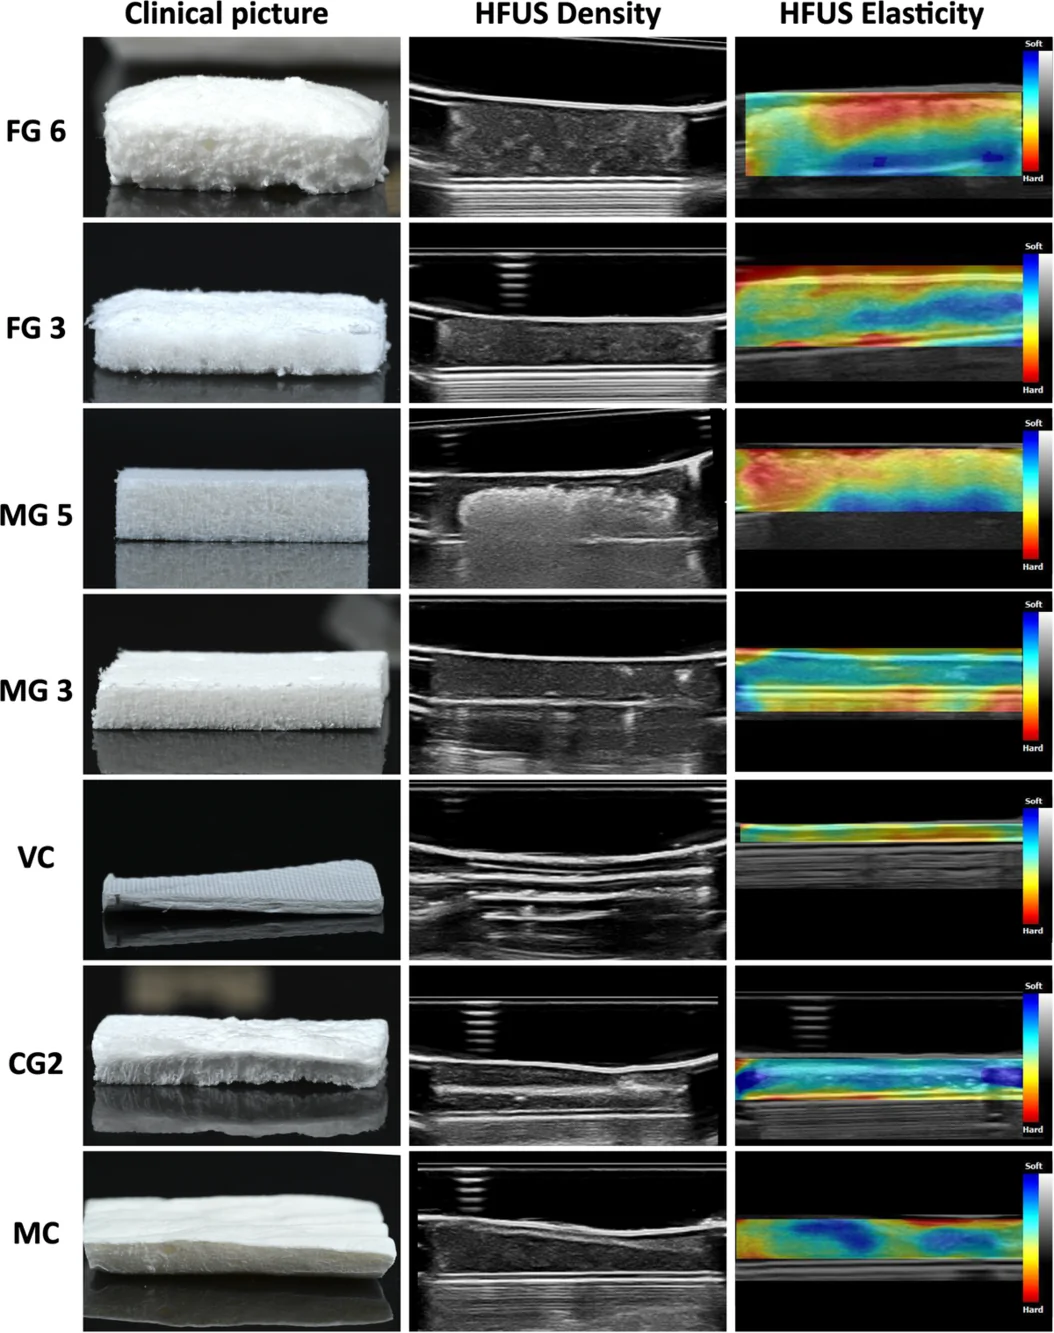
Representation of the different soft tissue substitutes, along with their ultrasonographic density and elasticity. (FG6) Fibro‐Gide 6 mm; (FG3) Fibro‐Gide 3 mm; (MG5) Mucogain 5 mm; (MG3) Mucogain 3 mm; (VC) Volumax; (CG2) Collagen Graft 2; (MC) Mucograft. The HFUS density showed, through the gray level scale, hypo‐ and hyperechoic regions, low gray levels indicating a low density of the material, while high gray scale showing a higher density. The elasticity showed clearly that during compression, the behavior of the different collagen matrices had different type of density and portion with hard and soft component.
